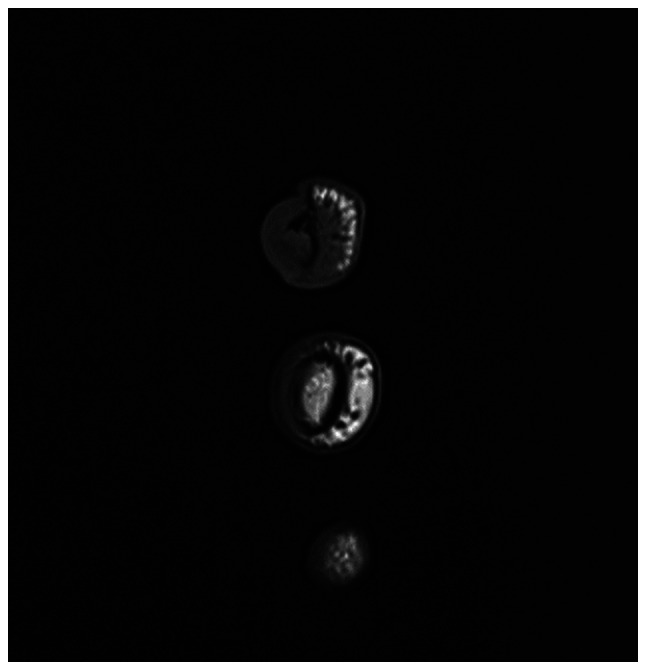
Axial T1-weighted magnetic resonance imaging scan demonstrating the lesion with low signal intensity.
